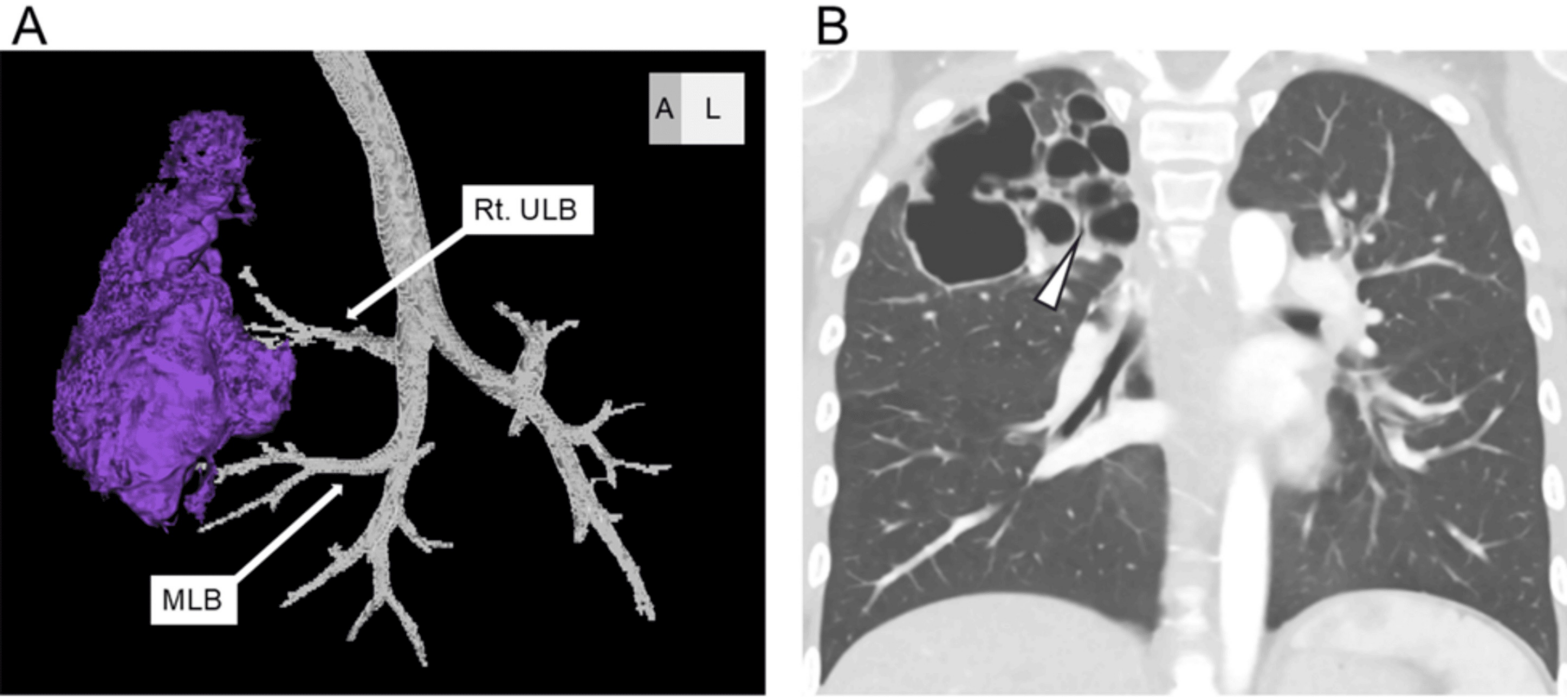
CT images of the cystic lesion (A) Three-dimensional reconstruction showing one of the abscesses extending across the right upper and middle lobes. (B) Coronal HRCT image demonstrating a large cyst with multiple clustered smaller cysts and a connection between the segmental bronchus and the cystic lesion (arrowhead) in the right upper lung field.
